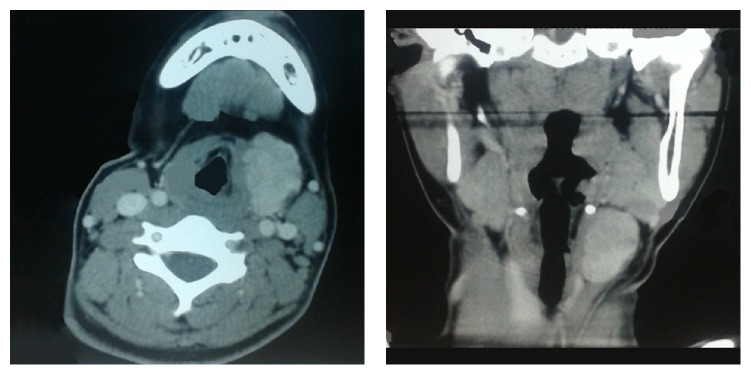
CT scan showing the soft tissue mass in the neck (axial and coronal view).
